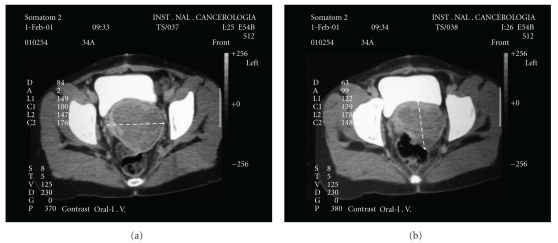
Contrast enhanced CT scan of the pelvis showing a solid cervical mass without evidence of direct involvement of bladder or rectum.
